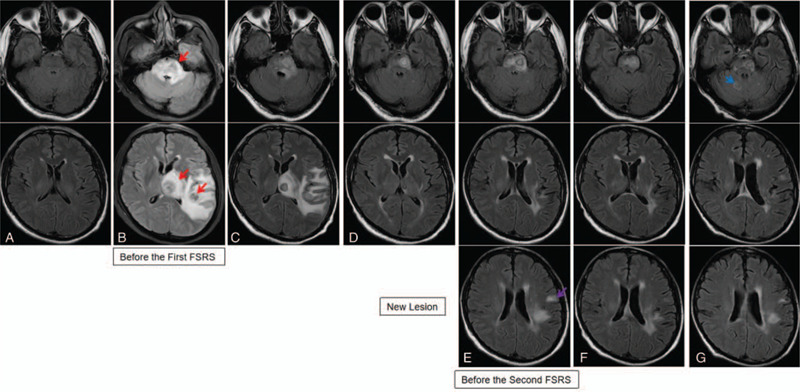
Cranial magnetic resonance imaging (MRI) scans revealed the sizes of brain metastases (BM) lesions and peritumoral brain edema (PTBE). (A) No metastatic lesion observed prior to surgery in March 2018; (B) 3 BM (red arrow indicates left temporal-parietal lobe, left thalamus lesions, and brain stem lesion), and large areas of PTBE observed in June 2018; (C) the BM lesions were reduced, and the area of the PTBE shrank progressively after radiation therapy; (D) all BM stayed reduced, and PTBE had disappeared in October 2018; (E) cranial MRI scans showed a new BM lesion in the left frontal lobe (purple arrow), and there were no obvious changes in the 3 BM in January 2019; (F) all BM lesions appeared further reduced in March 2019; (G) cranial MRI scans showed a new BM (blue arrow) in the cerebellum in October 2019.
